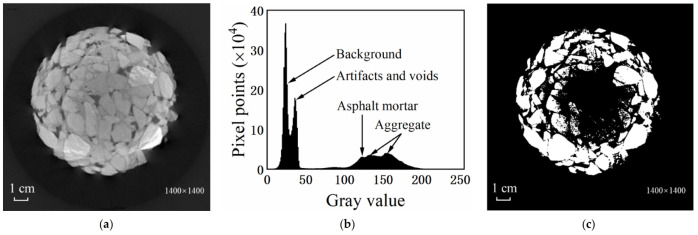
Characteristics of CT images. (a) Original image; (b) gray histogram; (c) global segmentation.
